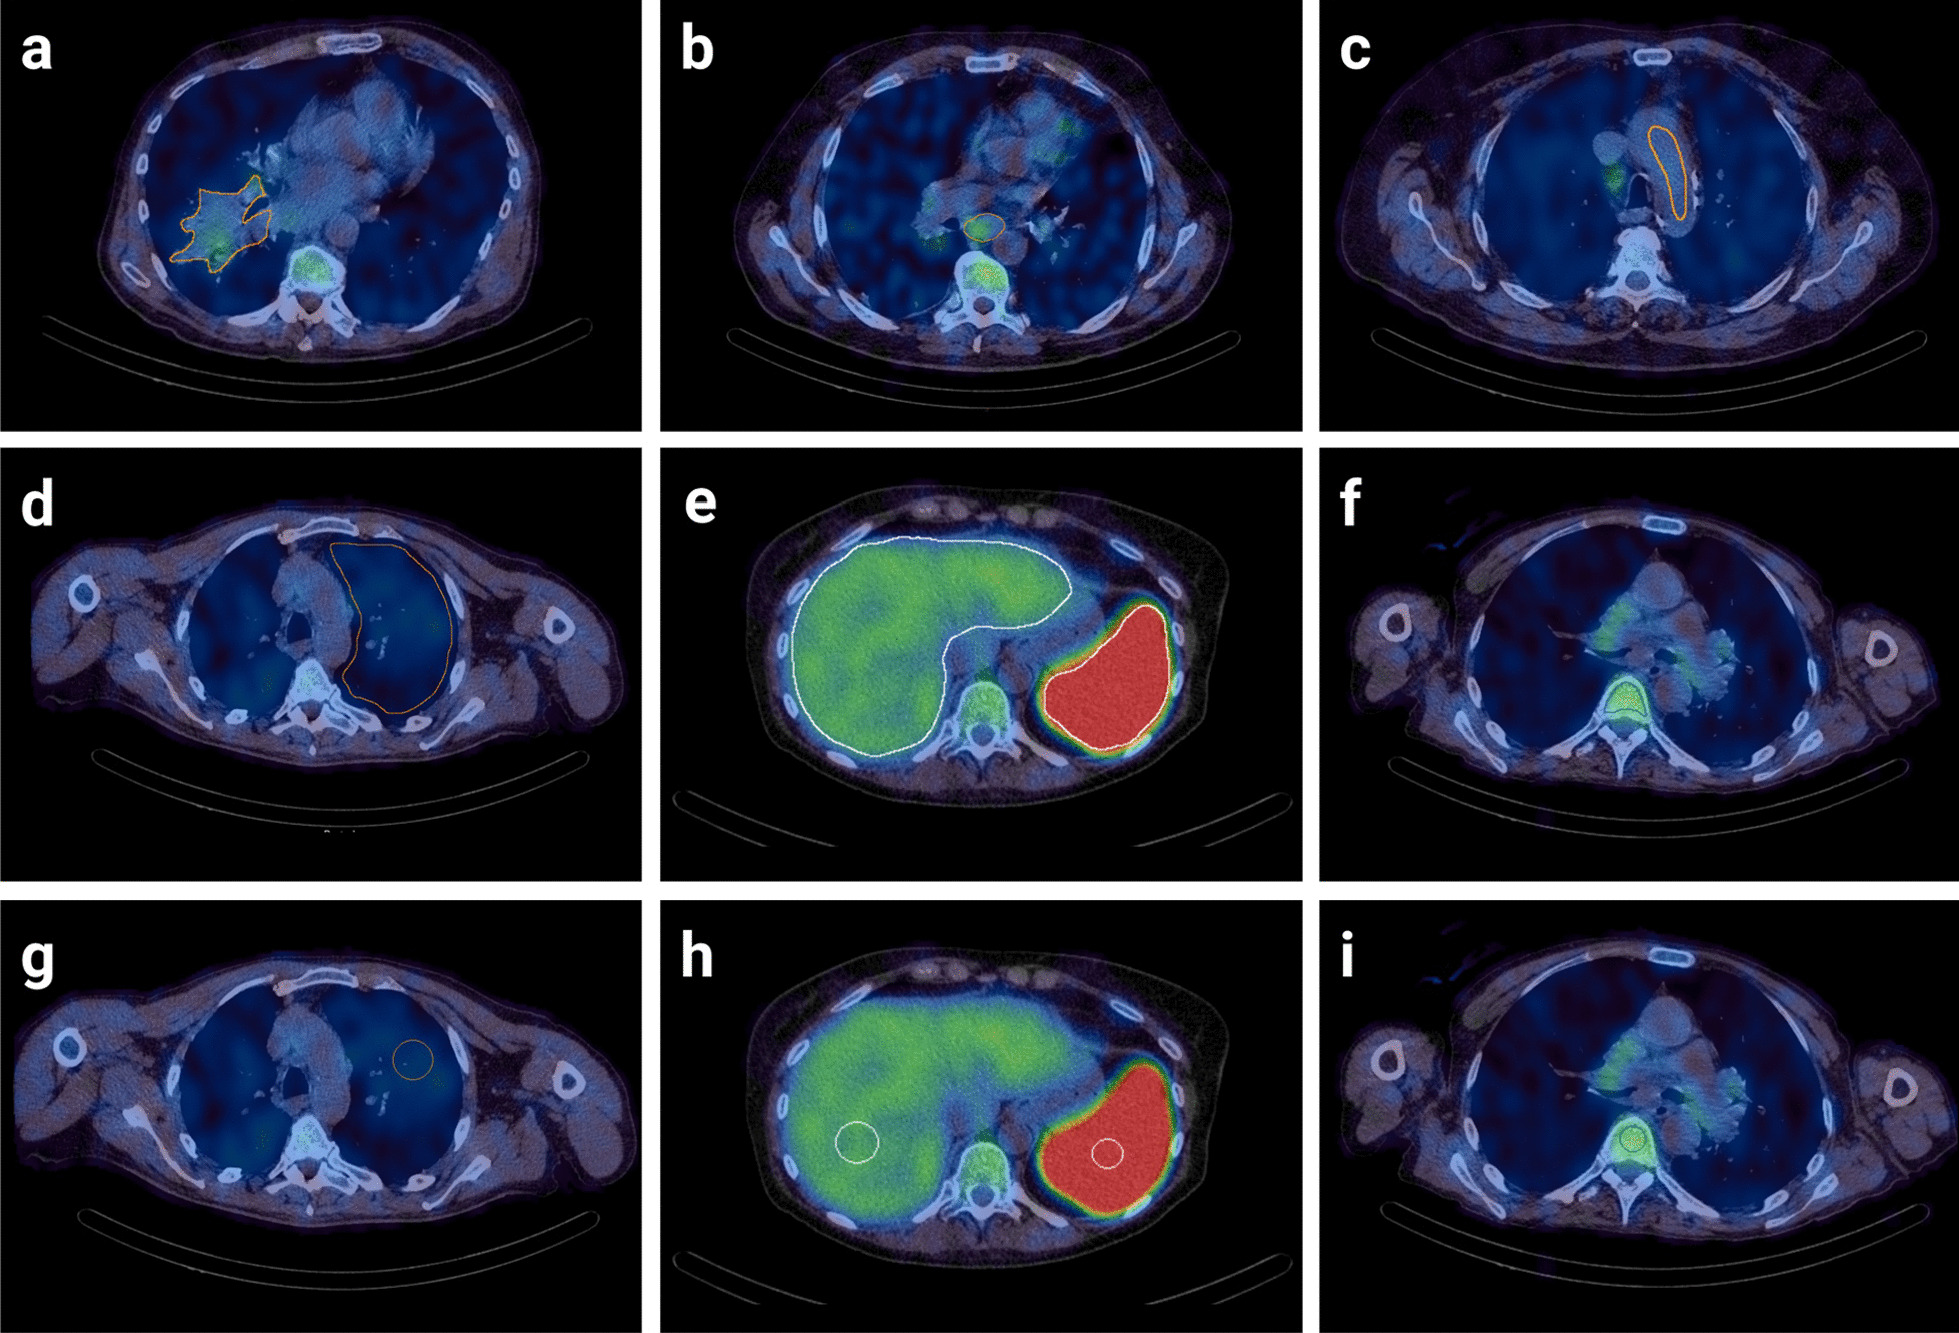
Image analysis using SUV_max_ scoring of [^99m^Tc]NM-01 SPECT/CT. a Freehand manual region of interest of a baseline (pre-treatment) primary right lower lobe tumour. b Freehand manual region of interest of a baseline station 7 (subcarinal) thoracic lymph node. c Blood pool SUV_max_ measured using a freehand manual technique. Healthy reference tissue SUV_max_ measurements determined by a freehand approach of d left upper lobe lung, e liver and spleen, and f bone marrow. Healthy reference tissue SUV_max_ measurements determined by volumetric rule-based approaches of g left upper lobe (3 cm sphere at level of aortic arch), h liver (3 cm sphere at level of the GOJ) and spleen (2 cm sphere), and i bone marrow (1.5 cm sphere at level of the carina)
